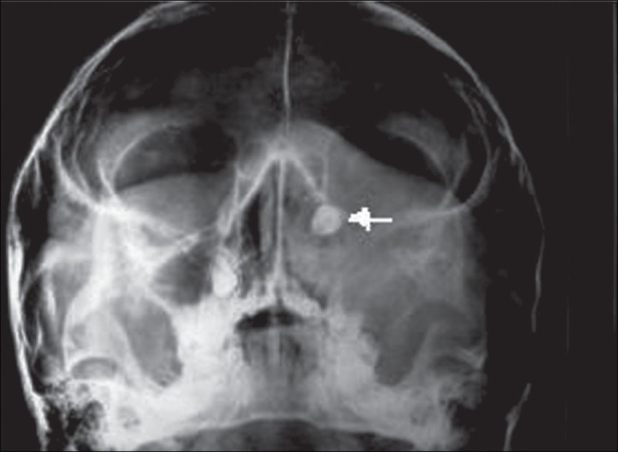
X-ray of the paranasal sinuses showing haziness of the left maxillary antrum with the dental structure near its roof (white arrow)
